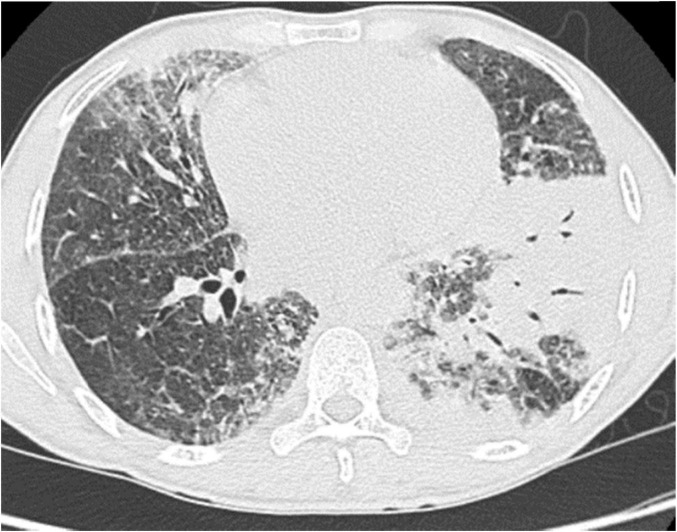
Chest CT scan showing chronic pulmonary consolidation in the left lower lobe.
